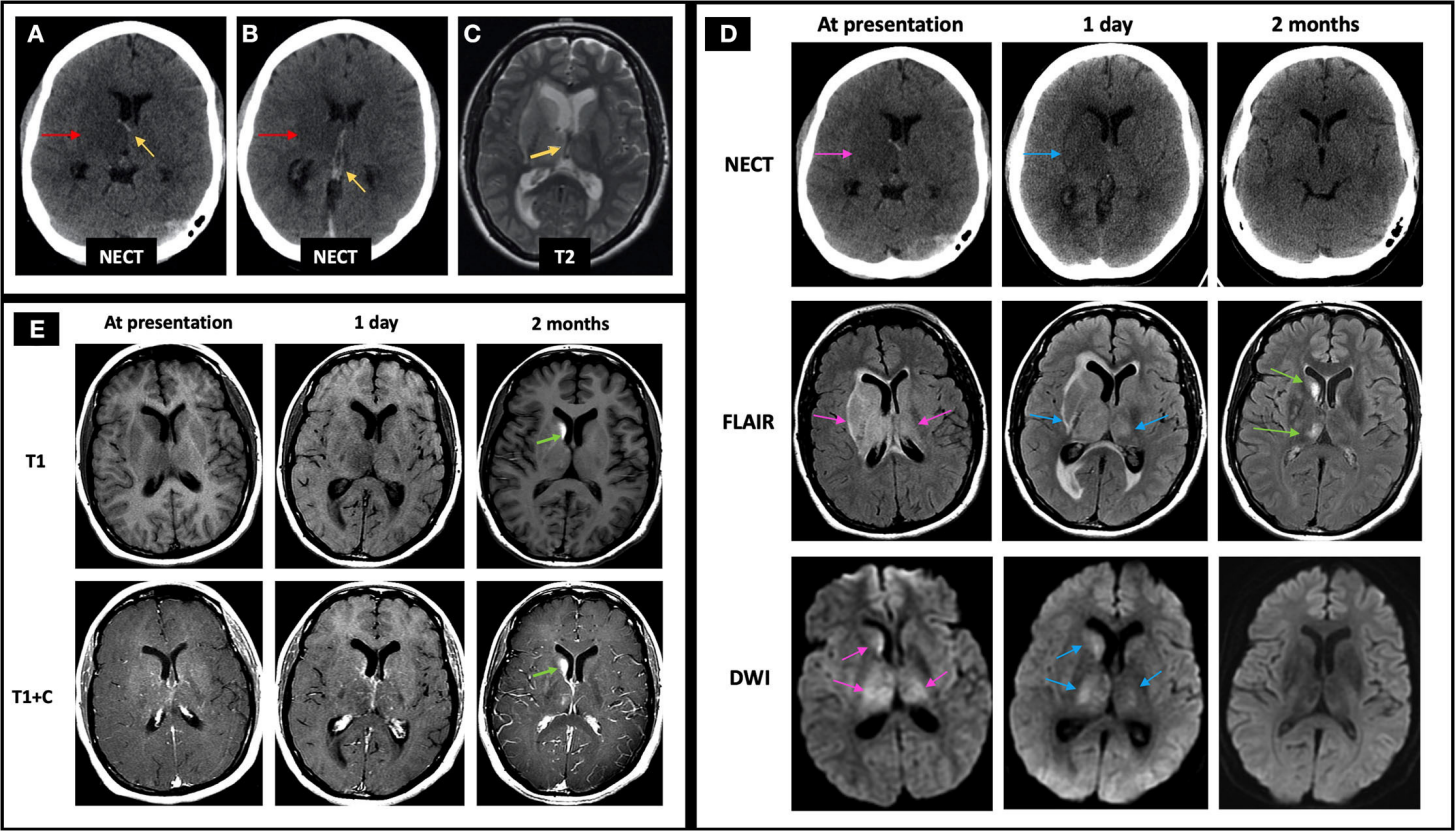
Case 4: A 43-year-old female presented with headaches, confusion, and left hemiparesis. Initial NECT showed curvilinear hyperdensity in the right greater than left ICVs (A,B, yellow arrows pointing to the ICVs, red arrows pointing to the adjacent edema) with associated T2 hypointensity on C-MRI (C, yellow arrow), suggestive of acute thrombus. FLAIR hyperintensity and diffusion restriction was seen at the right greater than left caudate, thalamus, and to a lesser degree the globus pallidus (D, left column, pink arrows) with mild increase in signal abnormalities 1 day later (D, middle column, blue arrows). At follow-up 2 months later, there is resolution of linear hyperdensity at the ICV (D, top row), as well as FLAIR and DWI hyperintensity at the right greater than left basal ganglia and thalamus (D, middle and bottom rows). Also, at 2 months follow-up imaging, the right caudate demonstrates encephalomalacia as evidenced by persistent FLAIR hyperintensity (D, middle row) and intrinsic T1 hyperintensity of the right caudate head on T1 pre-contrast images, compatible with pseudolaminar necrosis (E, green arrows). In (D), the pink arrows point to the edema and related signal abnormality from ICV thrombosis at time of presentation on NECT, FLAIR, and DWI, respectively (left column). The blue arrows in (D) point to the same areas of signal abnormality at 1 day follow-up (middle column). The right column in D demonstrates near resolution of signal abnormality on NECT, FLAIR, and DWI at 2 months follow-up with green arrows pointing to mild residual FLAIR hyperintensity at the right basal ganglia and thalamus. C-MRI, contrast-enhanced MRI; ICV, internal cerebral veins; NECT, non-contrast CT.
